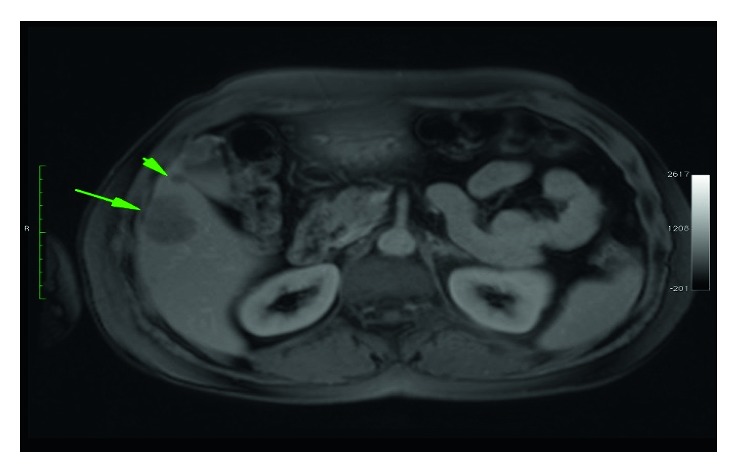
T1-weighted gradient-echo contrast-enhanced image. Two lesions are seen in the right liver lobe adjacent to the gallbladder.
